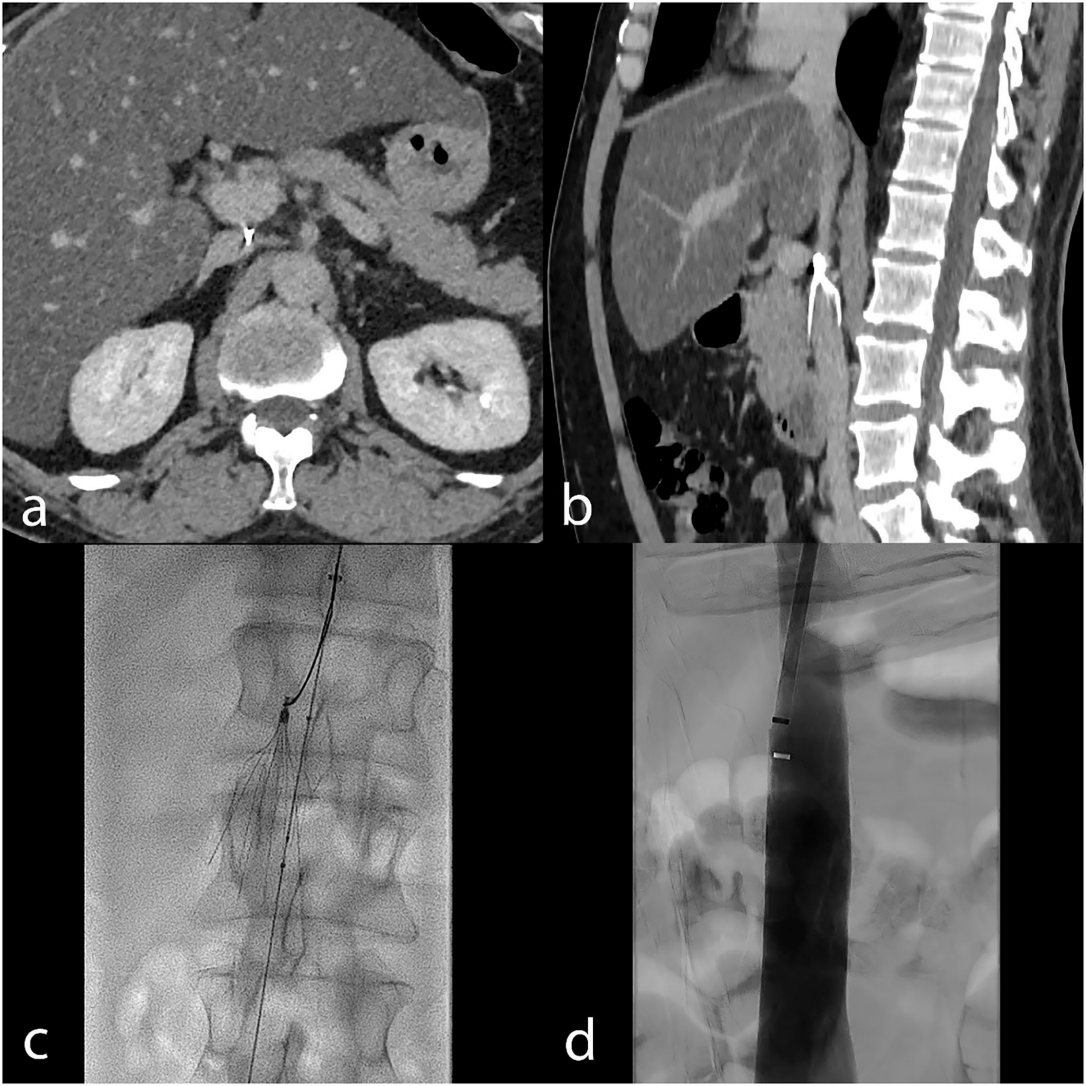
Embedded hook in the IVC wall is a common technical failure reason. CT venography in a patient with an embedded hook is shown on axial (a) and sagittal (b) images. In this case, advanced retrieval with the balloon displacement technique (periprocedural fluoroscopy image, (c) allowed removal. Final transcatheter subtracted cavography (d) shows the absence of complication.
